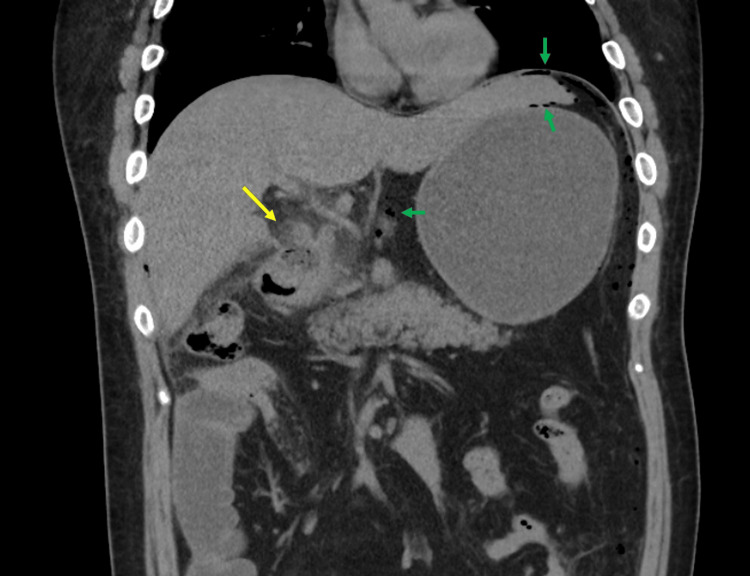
Abdominal computed tomography (CT) imaging. Abdominal CT shows a rupture of the intestinal wall and an increase in the CT value of the surrounding adipose tissue in the posterior upper wall of the duodenal bulb, suggesting a perforated duodenal ulcer (yellow arrow). Furthermore, free air is observed throughout the peritoneal cavity (green arrows).
